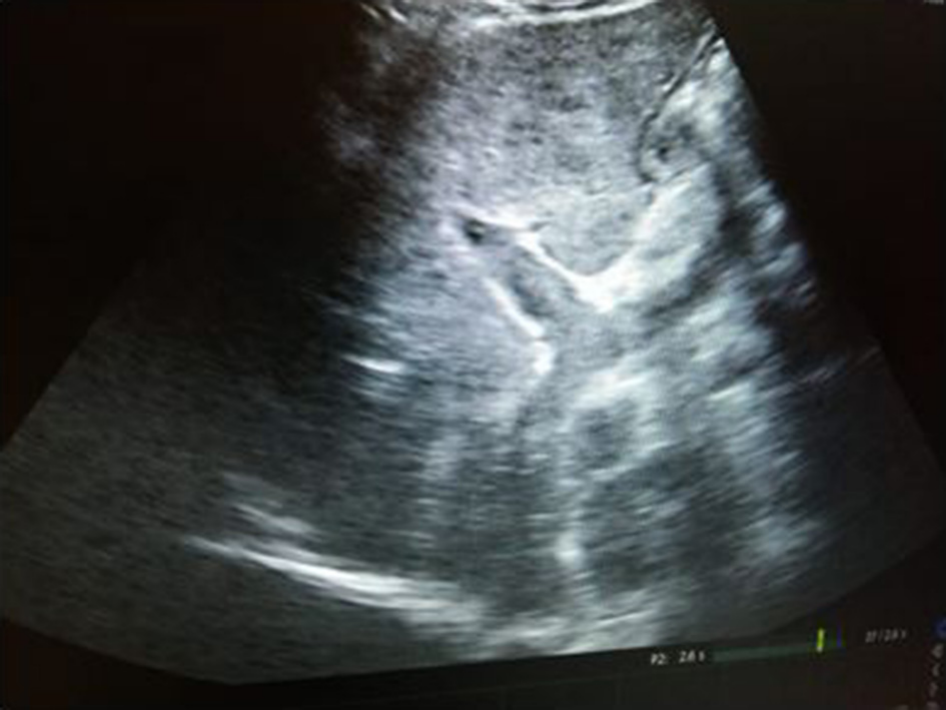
Ultrasound image of the right lobe of liver shows heterogeneous echotexture. Biopsy was taken from the heterogeneous appearing area of the right lobe of liver under ultrasound guidance.
